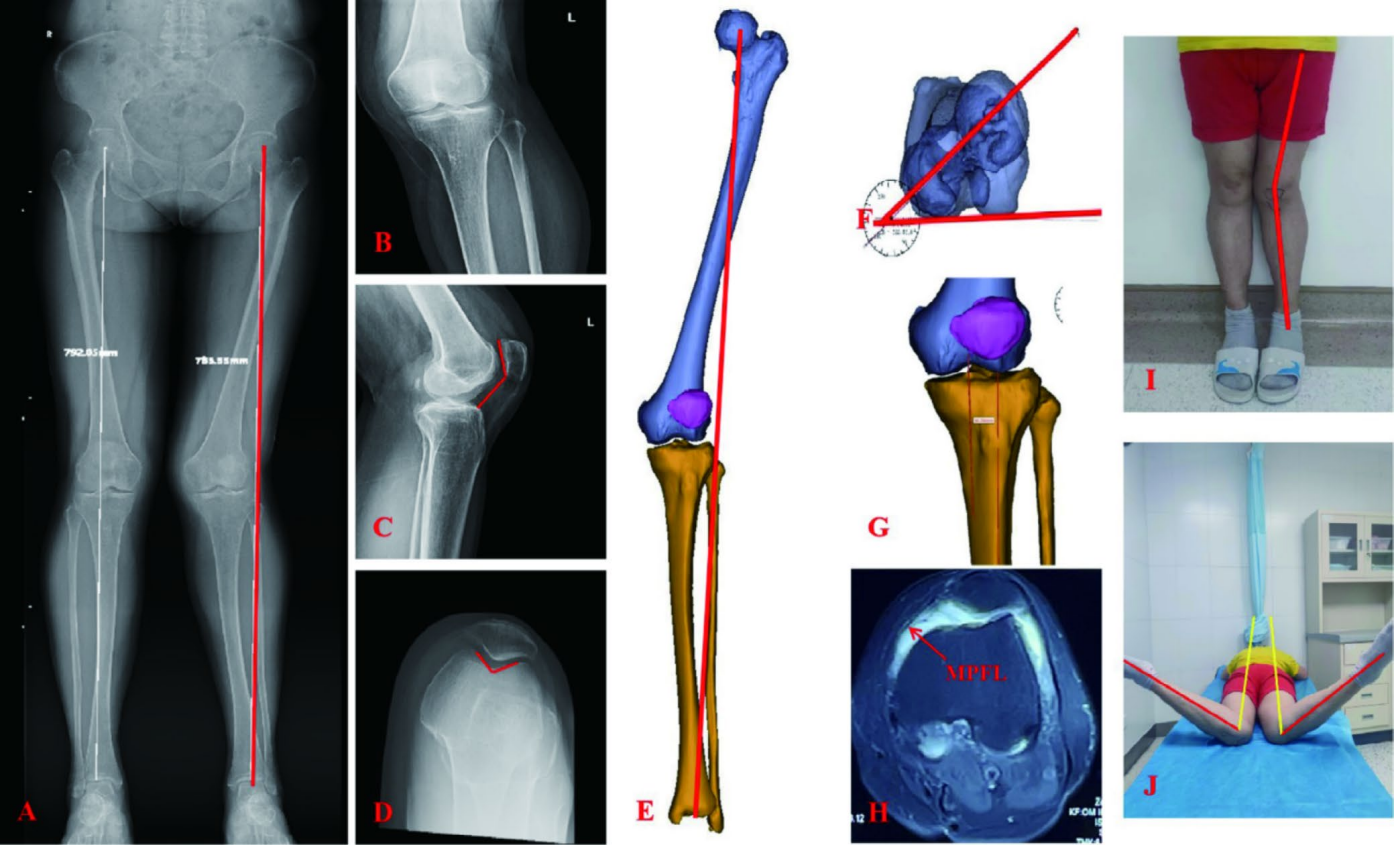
Preoperative photographs and fluoroscopy. (A) The preoperative standing X‐ray of the lower limbs. (B) The preoperative X‐ray of the knee in anteroposterior position. (C) The preoperative X‐ray of the knee in lateral position. (D) The preoperative X‐ray of the knee in 90° axial position. (E) The preoperative 3D‐CT revealed genu valgus of the left lower limb. (F) The preoperative 3D‐CT showed that the femoral anteversion angle was 37.4°. (G) The preoperative 3D‐CT showed that the tibial tuberosity‐trochlear groove distance was 16.8 mm. (H) The preoperative MRI revealed the injury of the MPFL. (I) Preoperative appearance revealed genu valgus of the left lower limb. (J) Preoperative appearance suggested that the left hip internal rotation angle was 70°.
